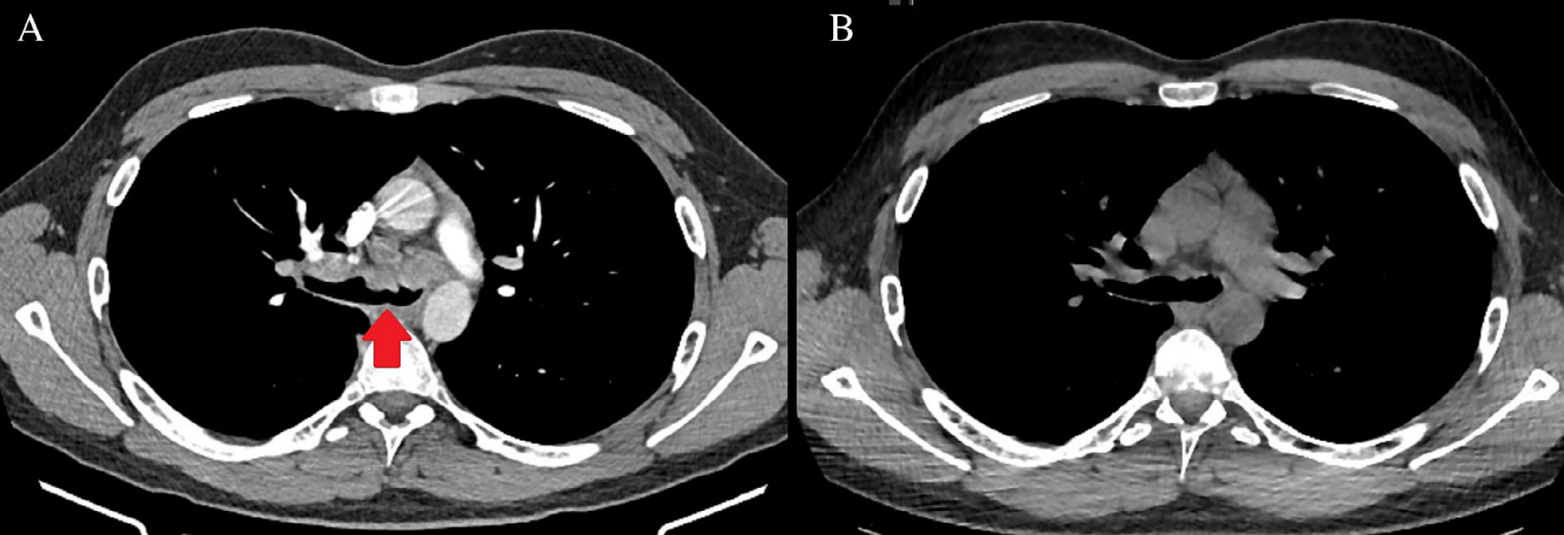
(A) Computed tomography (CT) chest demonstrating mediastinal lymphadenopathy and endobronchial involvement prior to tuberculosis (TB) treatment. (B) CT chest demonstrating significant improvement following six months of TB treatment.
